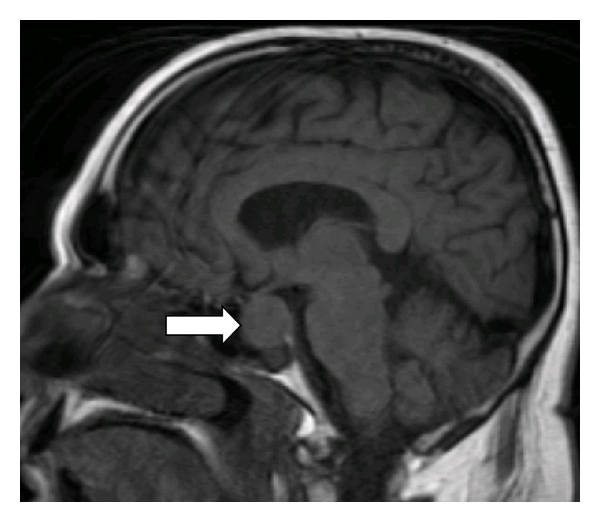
MRI of the brain showing pituitary macroadenoma compressing the optic chiasm.
